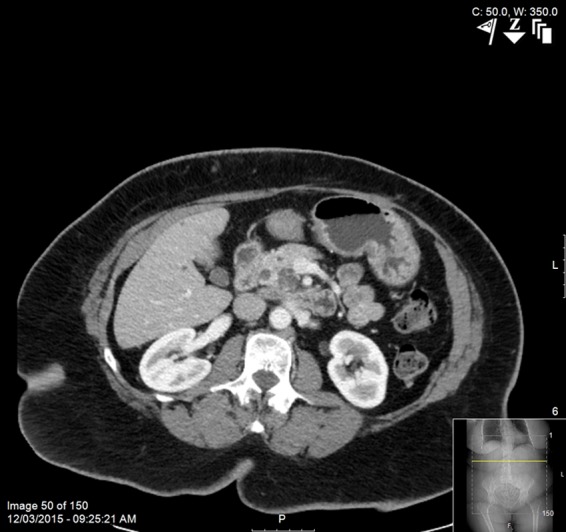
CT scan in 2015 showing stable uncinate cystic lesion and no associated mass.
